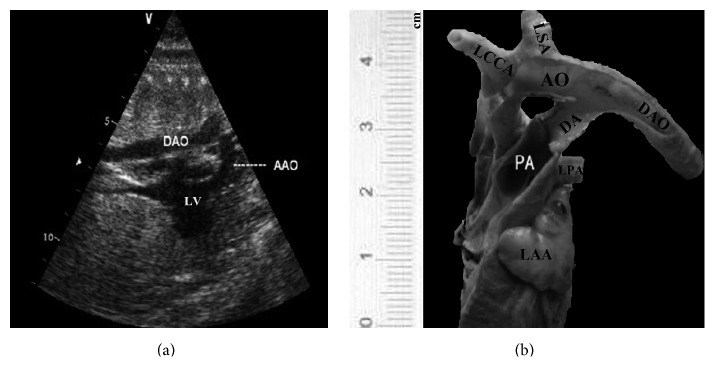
Patent ductus arteriosus (PDA) (38 w). (a) The long axis of aortic arch echocardiography section. (b) The long axis of aortic arch sectional anatomy. AAO: ascending aorta; LV: left ventricle; AO: aorta; DA: arterial duct; DAO: descending aorta, PA: pulmonary artery; LPA: left pulmonary artery; LCCA: left common carotid artery; LSA: left subclavian artery; LAA: left atrial appendage.
